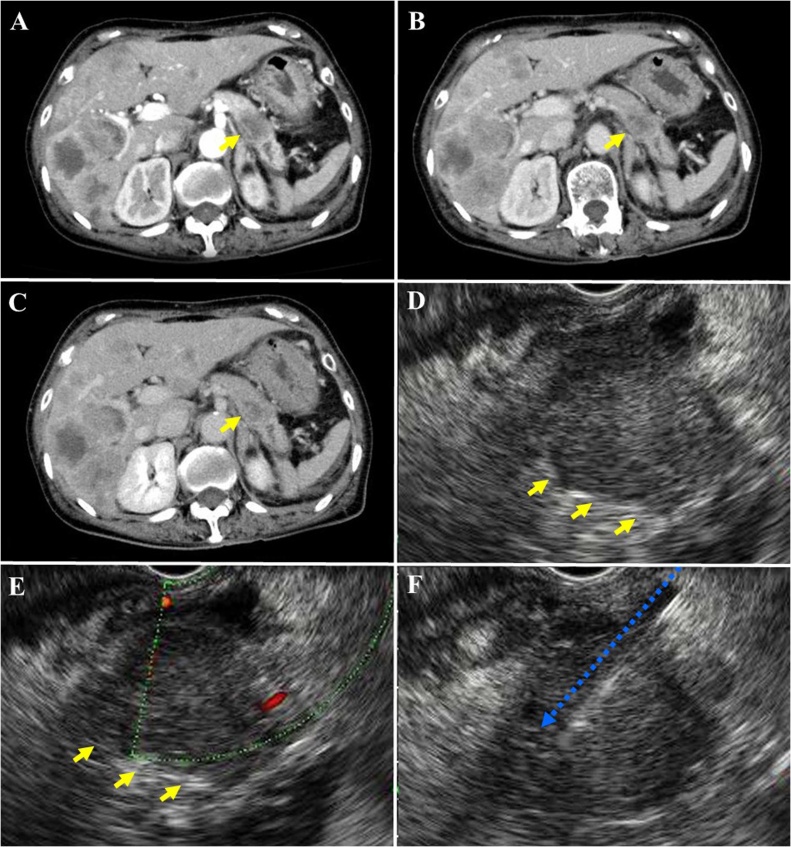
Findings of dynamic computed tomography and endoscopic ultrasound. Dynamic computed tomography revealed a hypovascular pancreatic tumor (yellow arrows) and multiple liver metastases (A–C), as did endoscopic ultrasound (E, F). Fine-needle aspiration was performed via the stomach (F, blue arrows).
